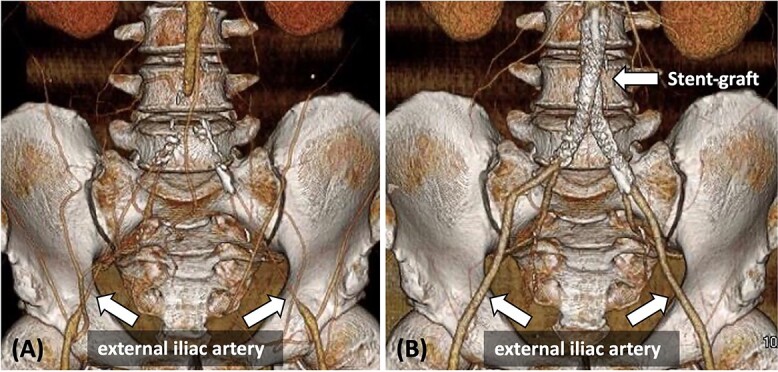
(A) Pre-operative contrast-enhanced CT reveals bilateral CIA occlusion from the abdominal aorta, with bilateral EIA stenosis, without calcification. (B) Contrast-enhanced CT 8 months post-surgery shows good bilateral EIA dilatation.
